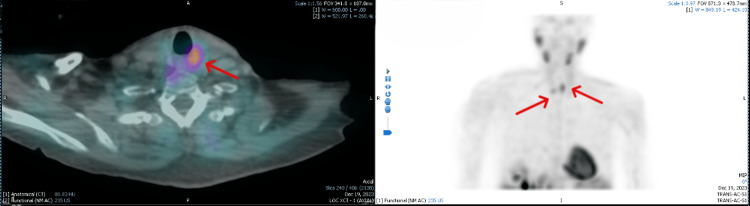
SPECT/CT demonstrating two foci of increased radiotracer uptake. Red arrows indicate the ectopic right superior parathyroid gland located in the paraesophageal region at the level of T1, and the left upper parathyroid gland posterior to the upper pole of the thyroid at C6-C7. Abbreviations: SPECT/CT: Single-Photon Emission Computed Tomography/Computed Tomography
